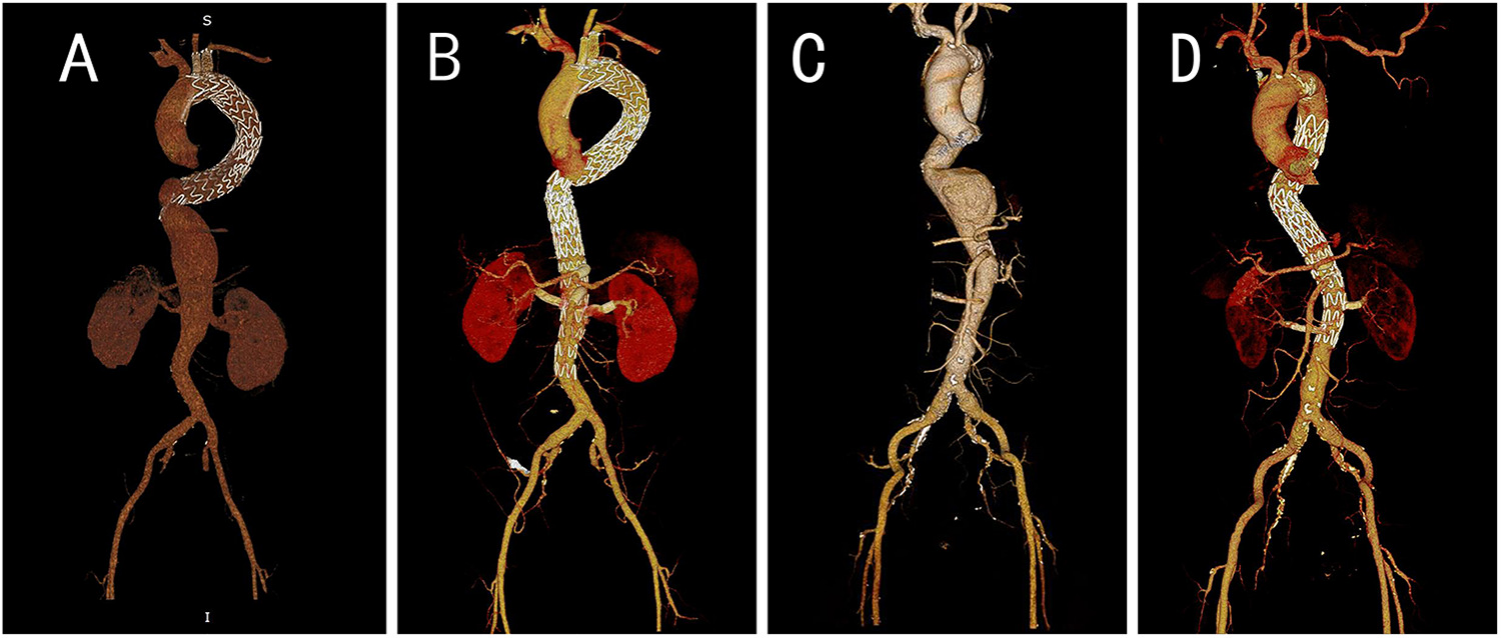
Pre- and post-operation CTA images for B EVAR and F EVAR. (A) Preoperative CTA of a patient with an abdominal aortic dissection aneurysm, revealing a giant false lumen, which led us to select B EVAR. (B) Postoperative CTA of the same patient. (C) Preoperative CTA of a patient with a thoracoabdominal aortic aneurysm, demonstrating an eccentric aneurysm, we opted for F EVAR. (D) Postoperative follow-up CTA of this patient.
